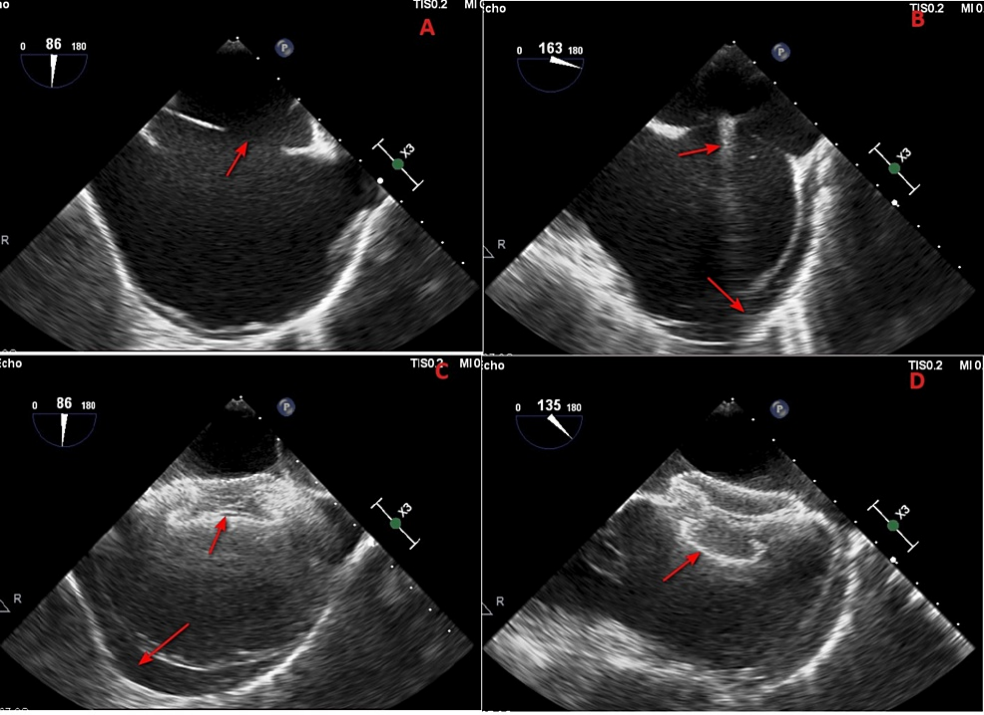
Transesophageal echocardiography around the intervention It shows the (A) eccentric location of the atrial septal defect (arrow), (B) appearance of the pericardial effusion before plugging the defect (arrows), (C) plugging of the defect with the device and progressing effusion (arrows), (D) proper deployment of the device with a significant pericardial effusion (arrow).
